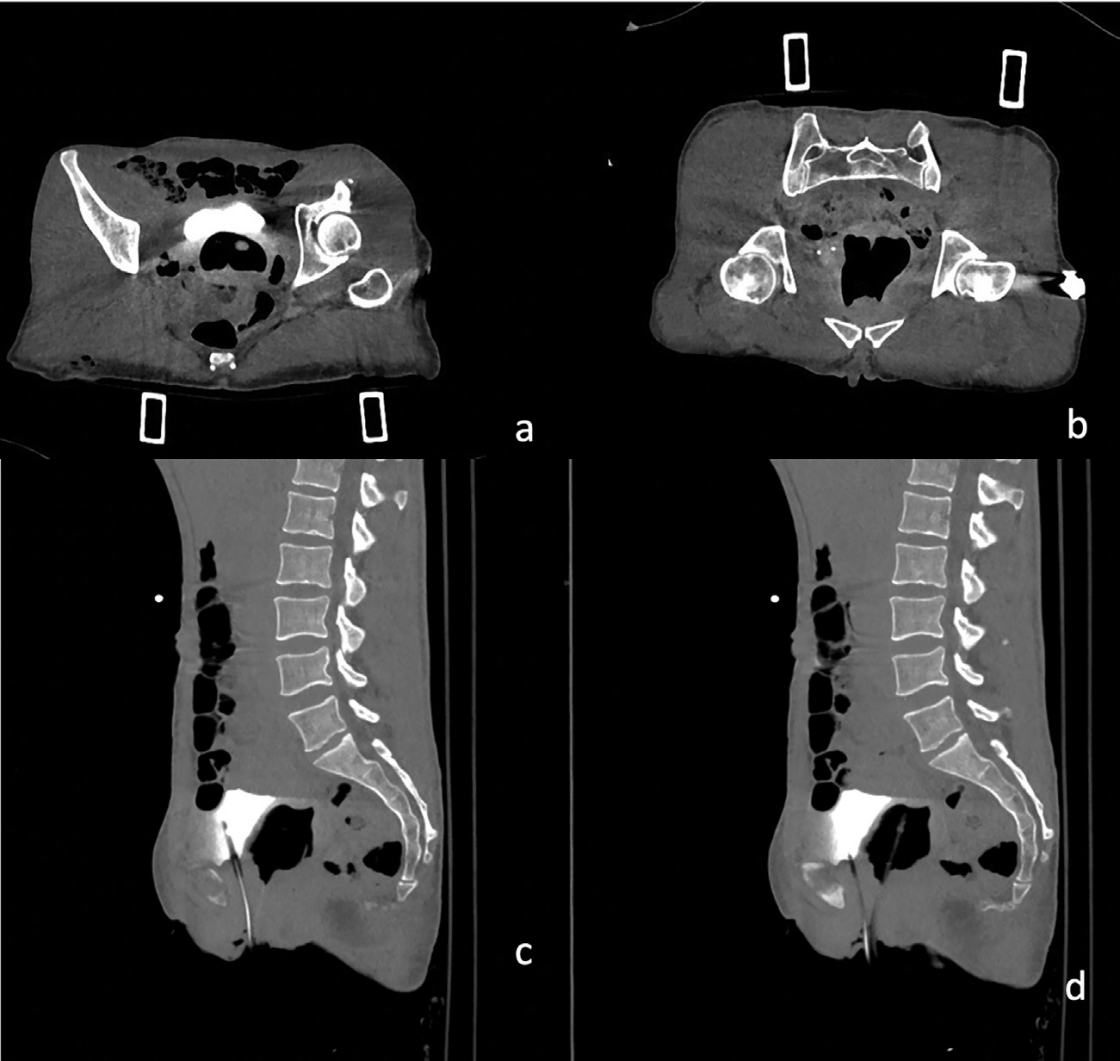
(A–D) CT axial and sagittal scan after the administration of Intravaginal positive contrast medium through a vaginal catheter demonstrates the extravasation of air and contrast confirming the diagnosis of a vaginal wound.
